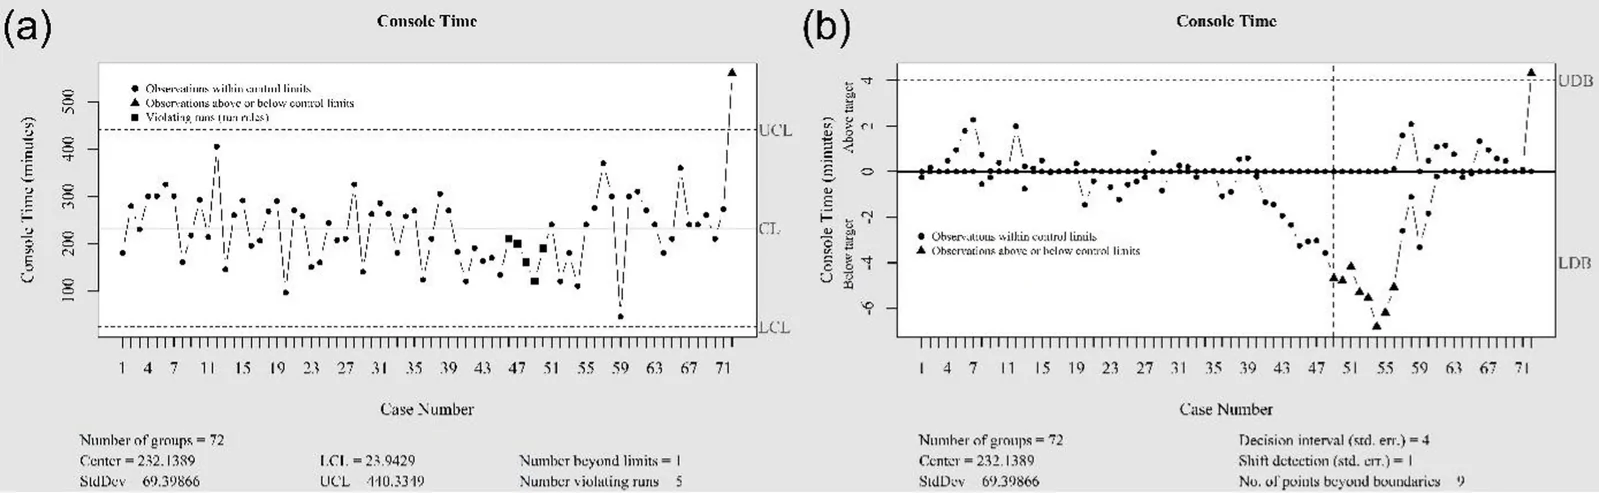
(a) Console Time (CT) against case number. (b) Console Time (CT) CUSUM chart. *UDB* Upper Decision Boundary, *LDB* Lower Decision Boundary, *UCL* Upper Control Limit, *CL* Center Line, *LCL* Lower Control Limit, *StdDev* Standard Deviation, *Std.err.* Standard Error
